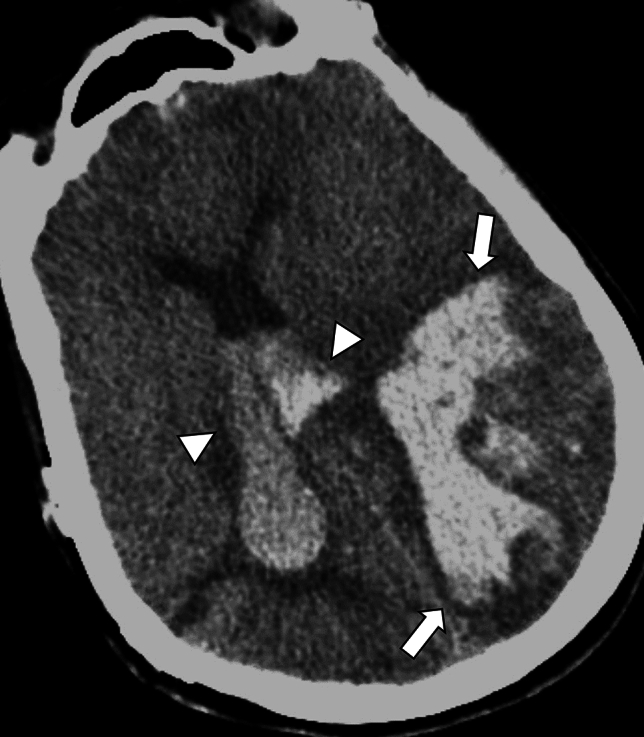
A 60-year-old male with probable CAA with supporting pathology. CT shows left temporo-occipital hemorrhage with finger-like projection (arrows), extending both of the lateral ventricles (arrowheads)
